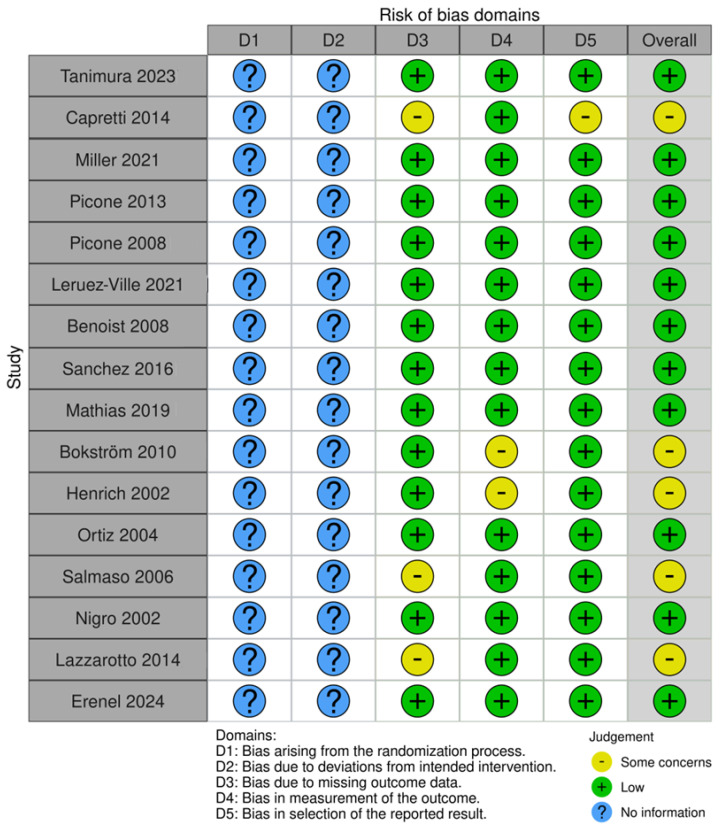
Risk bias of all studies with ultrasound anomalies.
